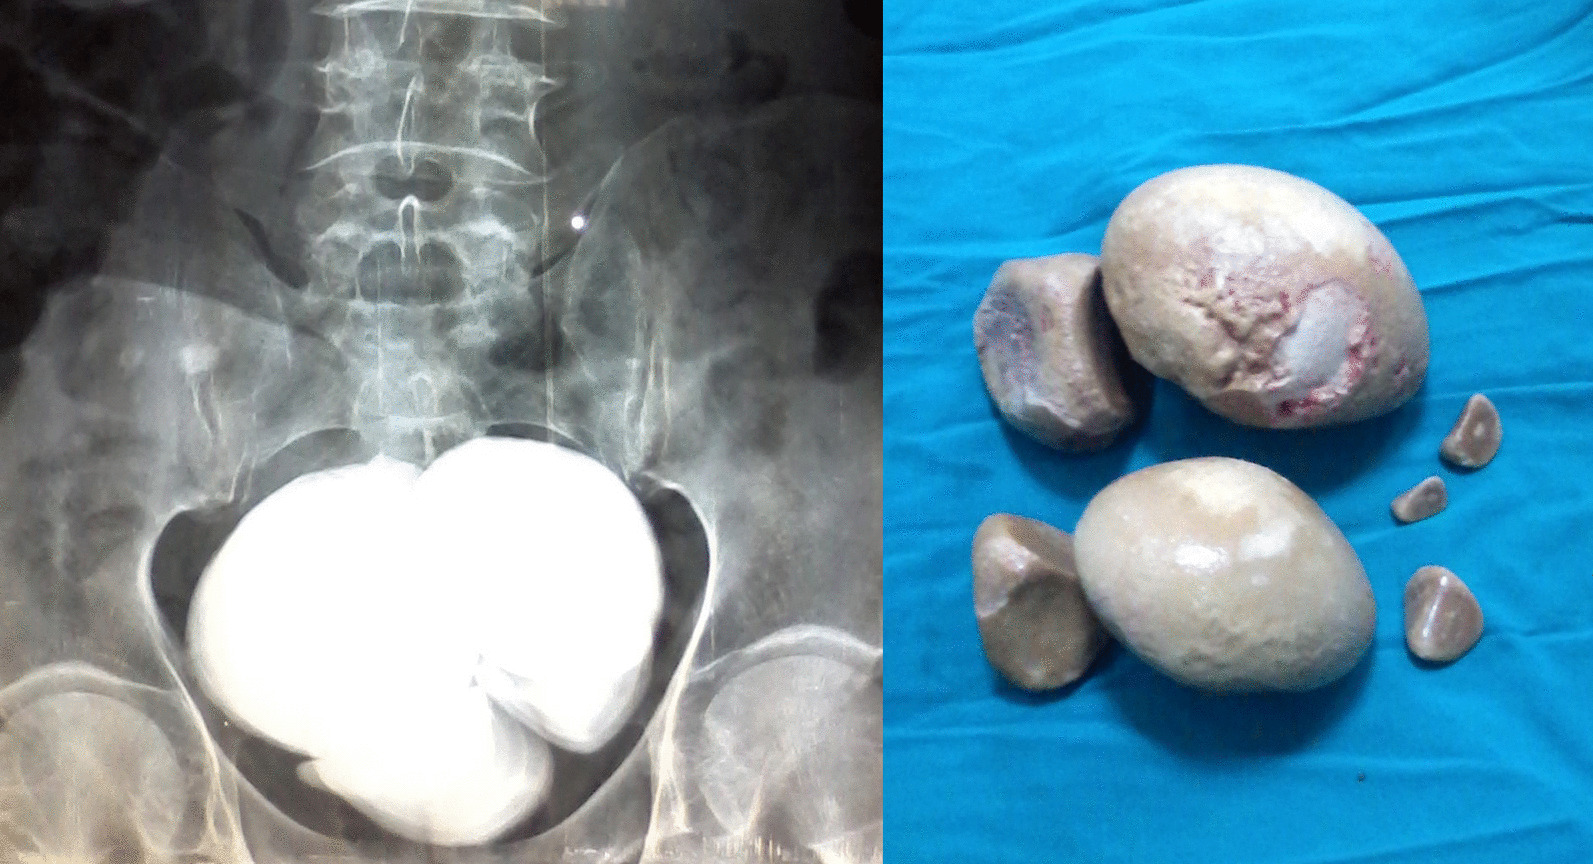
Multiple giant stones of the urinary bladder in a patient with neurogenic voiding dysfunctions: The left-sided figure is a plain radiography showing dense overlapping bladder stones. The right-sided figure shows the gross appearance of these stones after cystolithotomy
